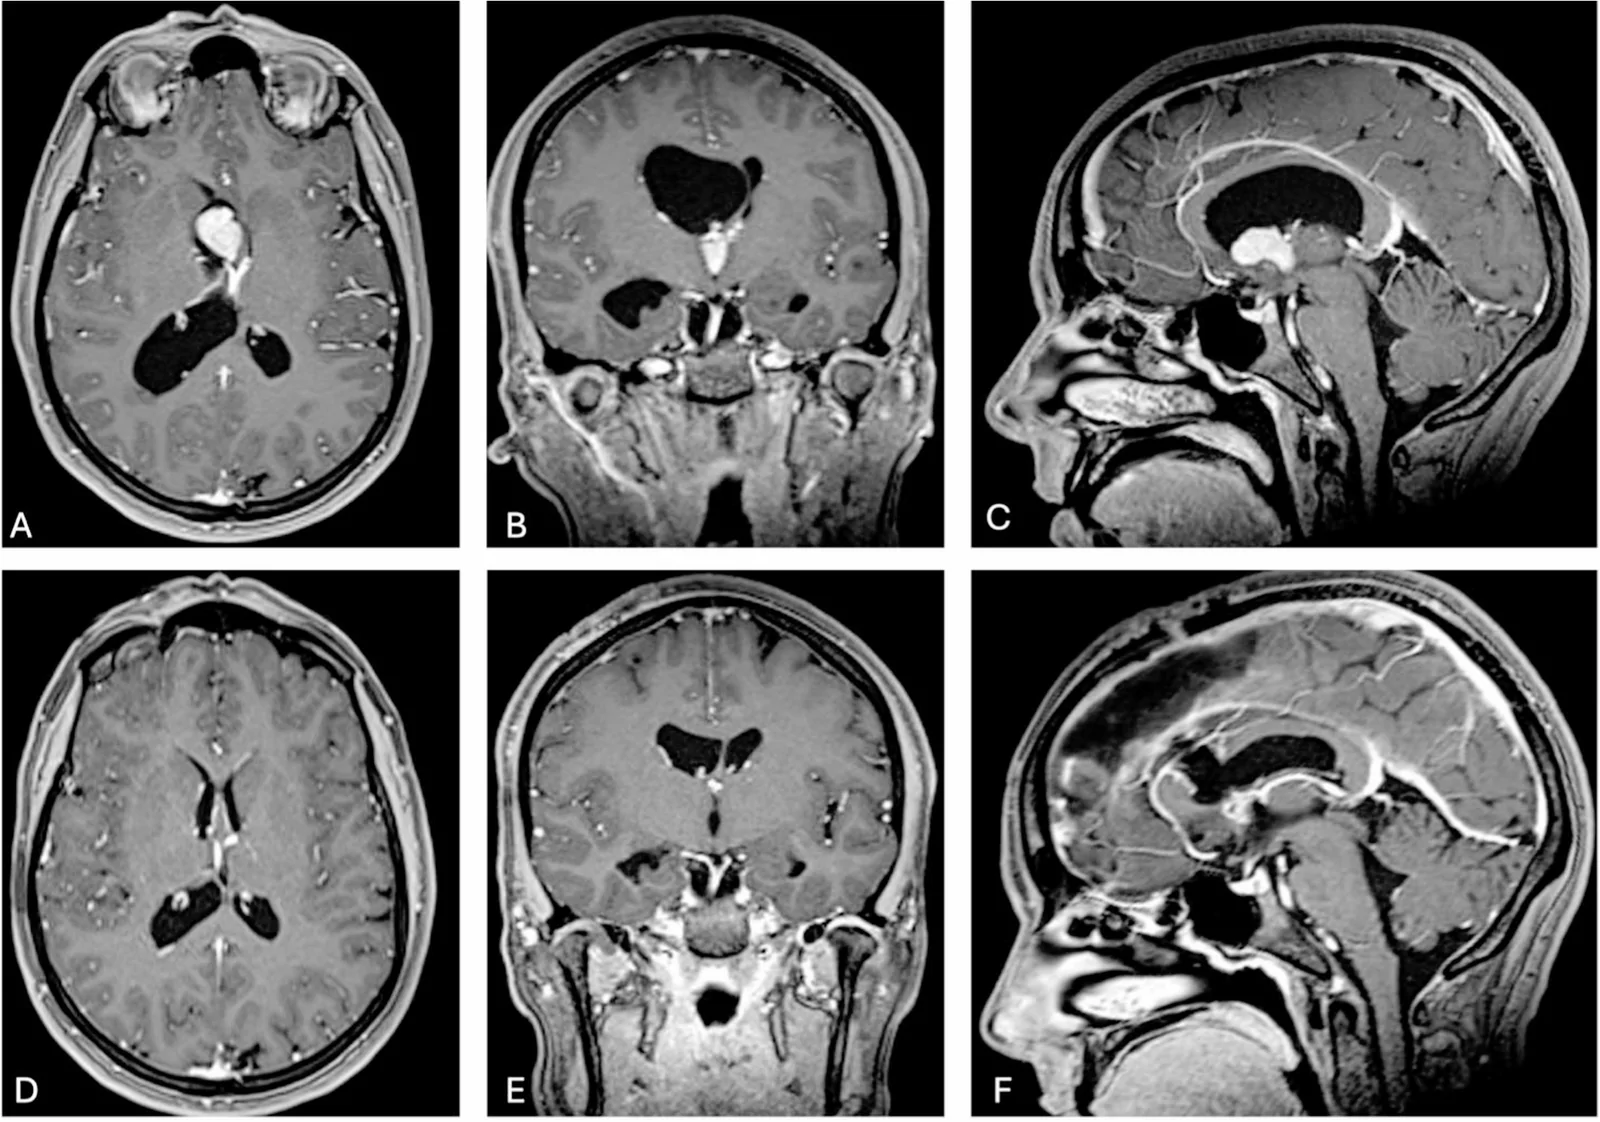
Preoperative brain MRI, axial (A), coronal (B) and sagittal (C) sequences. The lesion in the right lateral ventricle and involving the third ventricle, caused an expansive effect on the adjacent right ventricular middle cell with contralateral displacement of the pellucid septum. Signs of transependymal cerebrospinal fluid transudation were also present. Postoperative brain MRI at last follow-up, axial (A), coronal (B) and sagittal (C) sequences. Gross-total lesion removal and no regrowth were evident
